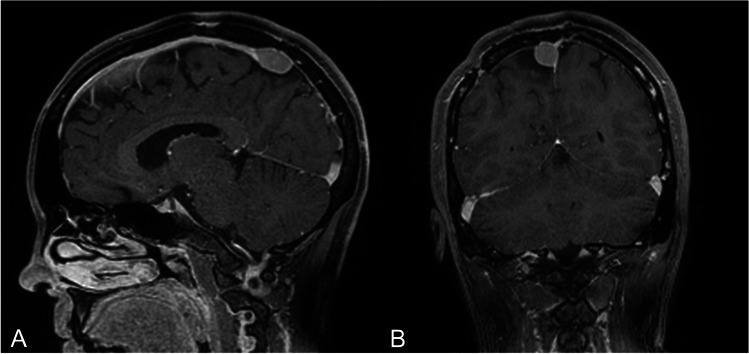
Contrast-enhanced brain MRI, sagittal (A) and coronal (B) TI-weighted sequences: recurrence of a right parasagittal rolandic meningioma treated by Simpson grade III resection with narrowed superior sagittal sinus > 50%
